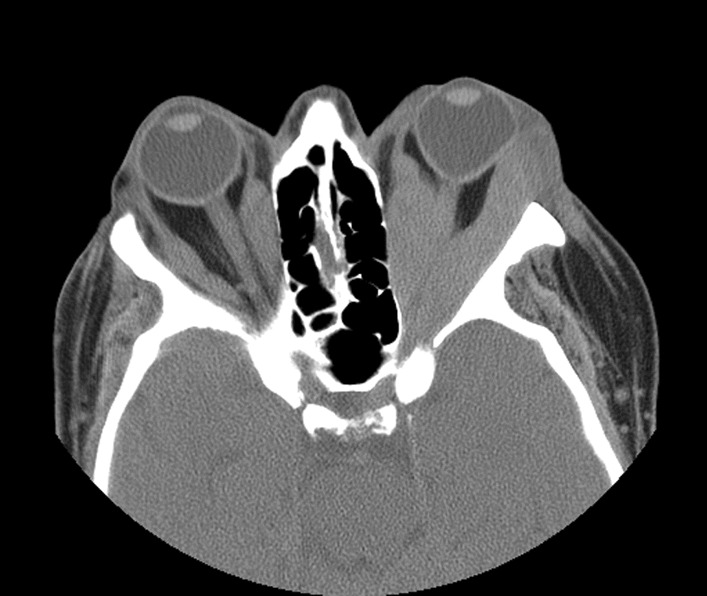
Orbital computed tomography (coronal)
